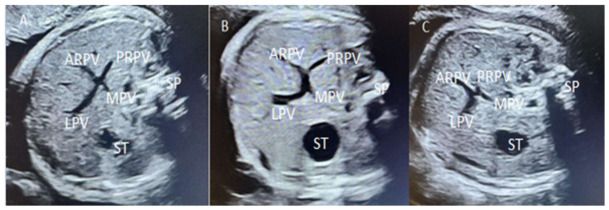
Types of fetal intrahepatic umbilical–portal venous anastomoses on transverse abdominal ultrasound. Panels correspond to (A) T-shaped, (B) X-shaped, and (C) H-shaped configurations. ARPV, anterior right portal vein; PRPV, posterior right portal vein; MPV, main portal vein; LPV, left portal vein; SP, spine; ST, stomach.
